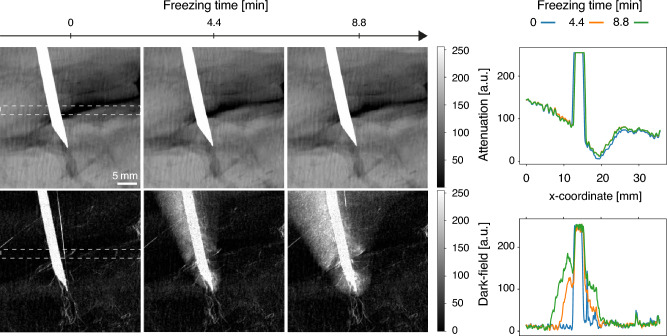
Series of radiographs (left) and line plots (right) of a pork neck slab frozen using a copper rod connected to a liquid nitrogen reservoir on one end. While the extent of the frozen region is clearly visible in the dark-field images, it is not visually detectable in the attenuation images. The line plots show the signal change over time in the dotted white rectangular region, averaged in the vertical direction.
